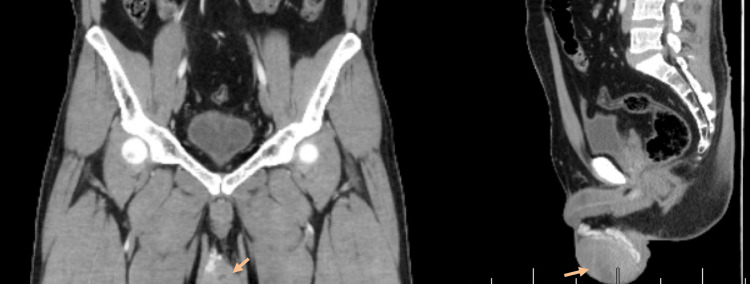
A pelvic CT (coronal and sagittal view) revealing a heterogeneous enlarged right testes with central foci of hypodensity.
